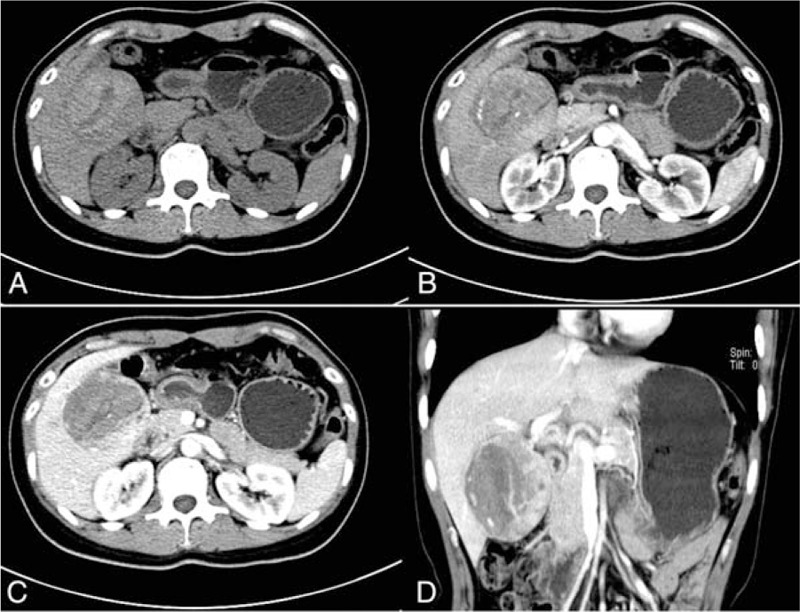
Computed tomography (CT) images of the tumor. A, Plain CT scan showed a round mixed density lesion with well-demarcated margin in the segment V. B, Contrast-enhanced CT showed a marked inhomogeneous enhanced lesion in the arterial phase. C, Contrast CT scan of the same lesion showed the lesion still enhanced obviously in the portal phase. D, Three-dimensional reconstruction of the liver mass on CT.
